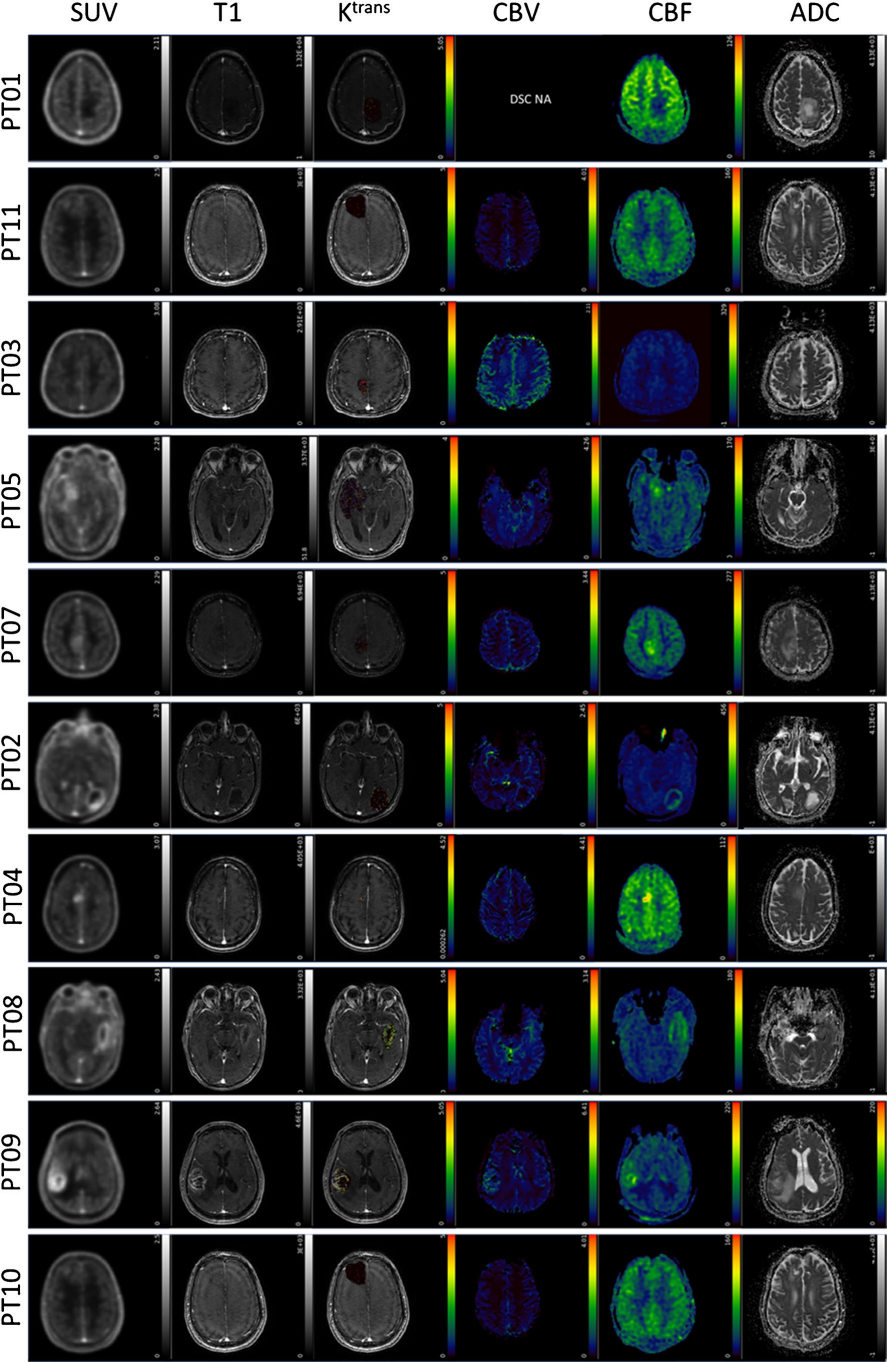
FPIA PET/MRI images in patients with grades II, III and IV gliomas. Axial PET and MRI images for grade II (PT01 and PT11), grade III (PT03, PT05, PT07) and grade IV (PT02, PT04, PT08, PT09, PT10) glioma patients including standardised uptake value for the last 5 time frames (SUV) from FPIA PET, T1 weighted MRI sequence, dynamic contrast-enhanced MRI (DCE)-contrast agent plasma/interstitium transfer rate constant (Ktrans), dynamic susceptibility contrast (DSC) MRI-cerebral blood volume (CBV), arterial spin labelling MRI-cerebral blood flow (CBF) and diffusion-weighted imaging MRI-apparent diffusion coefficient (ADC)
